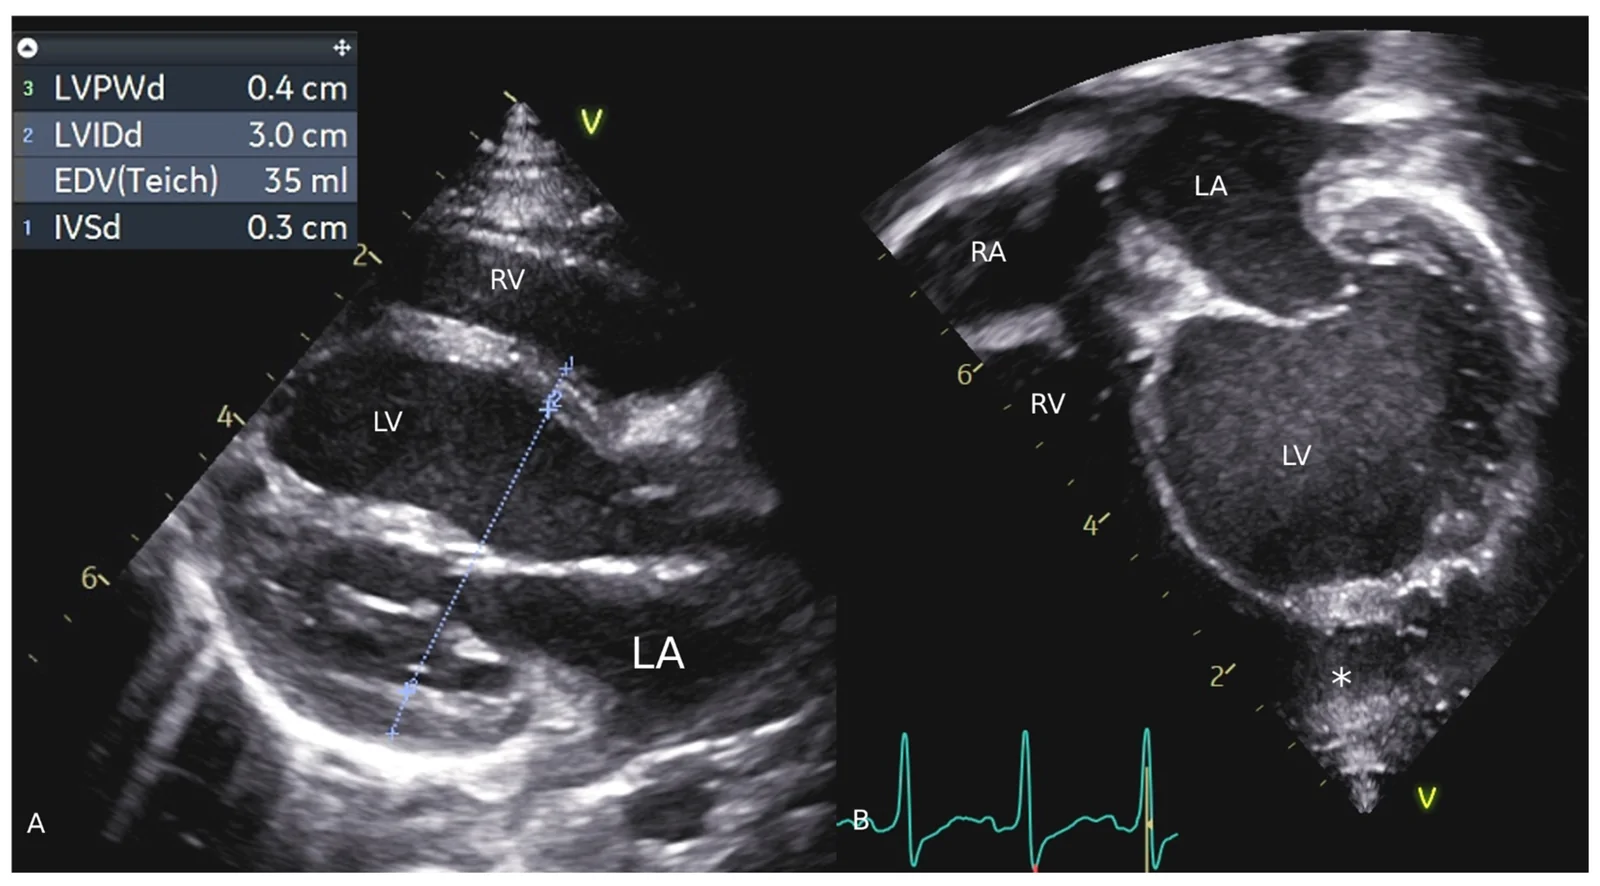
(A) Parasternal long-axis (PLAX) and (B) apical four-chamber views showed the hallmark morphological feature of ILVAH: a blunt and truncated, spherical geometry of the left ventricular apex, while the right ventricle showed a “banana-shaped” (denoted by *) elongated geometry wrapping around the distal left ventricle.
